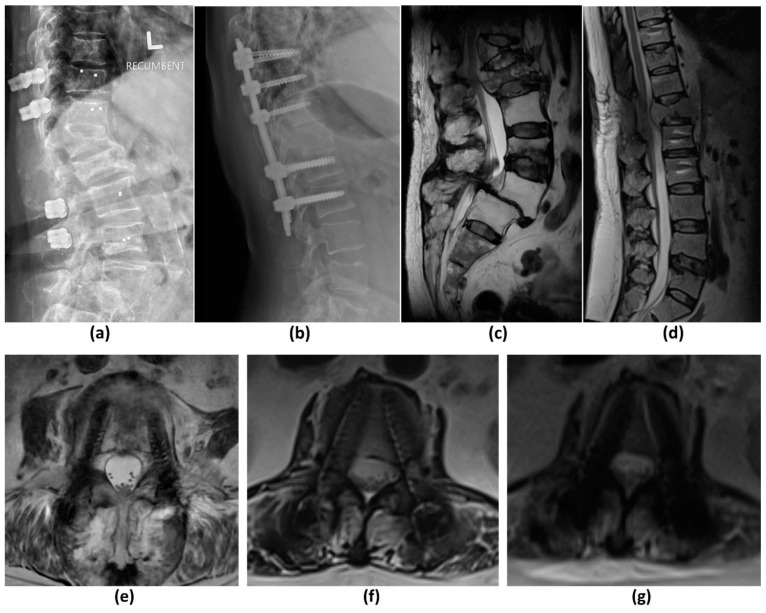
Lateral radiograph (a), sagittal (c) and axial (e) T2-weighted metal artifact reduction sequence (MARS) MR image of a patient with CFR-PEEK screw implants in the spine. Lateral radiograph (b), sagittal (d) and axial (f) T2-weighted MARS (“WARP”) MR image of a patient with titanium screw implants in the spine. The titanium screws are partially visualized in (d) due to the slice orientation and are best seen in the T9, L1 and L2 vertebral bodies. In contradistinction to the titanium implants, CFR-PEEK implants are radiolucent on radiographs and result in less metal-related artifacts on MRI. Axial T2-weighted image of the patient with titanium implants without MARS demonstrate significantly increased geometric distortion and signal losses, rendering assessment of the vertebral body and contents of the spinal canal difficult (g).
